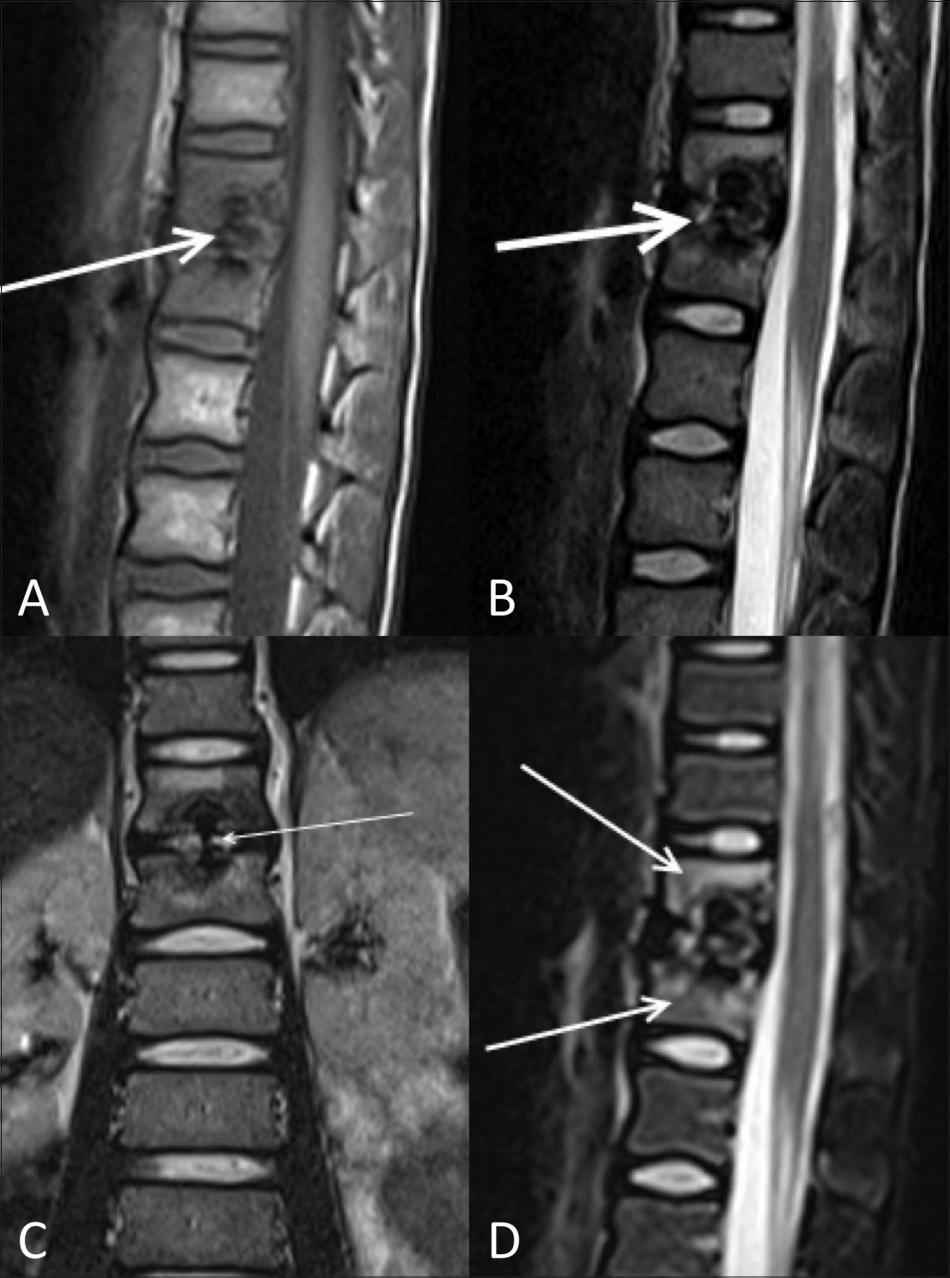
Sagittal T1W (A), sagittal T2W (B), and coronal T2W (C) MRI images show swelling of the nucleus pulposus with hypointense signal (arrows) on both the T1W and T2W images, due to calcification. The coronal T2W image (C) clearly shows the dumbbell-shaped calcification with intravertebral herniation without end plate destruction (arrow). Sagittal STIR MRI image (D) shows evidence of marrow edema (arrows) in the bodies of the adjacent vertebrae
